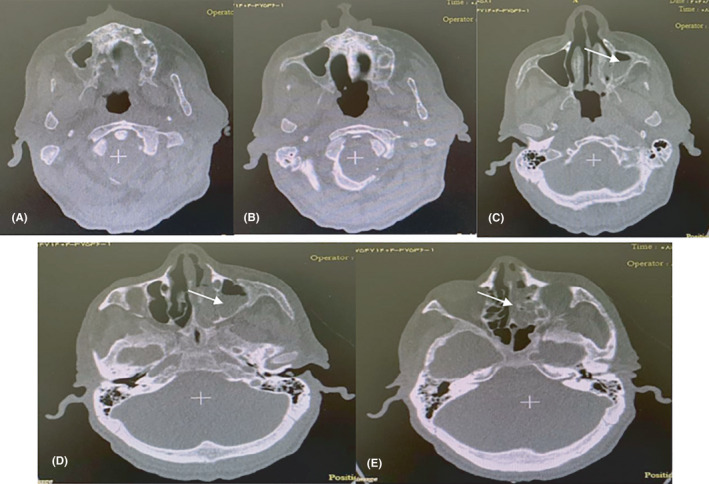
sinus CT Scan. On the first day of hospitalization pan‐sinusitis left sinuses (turbidity of maxillary and ethmoid left sinuses)
